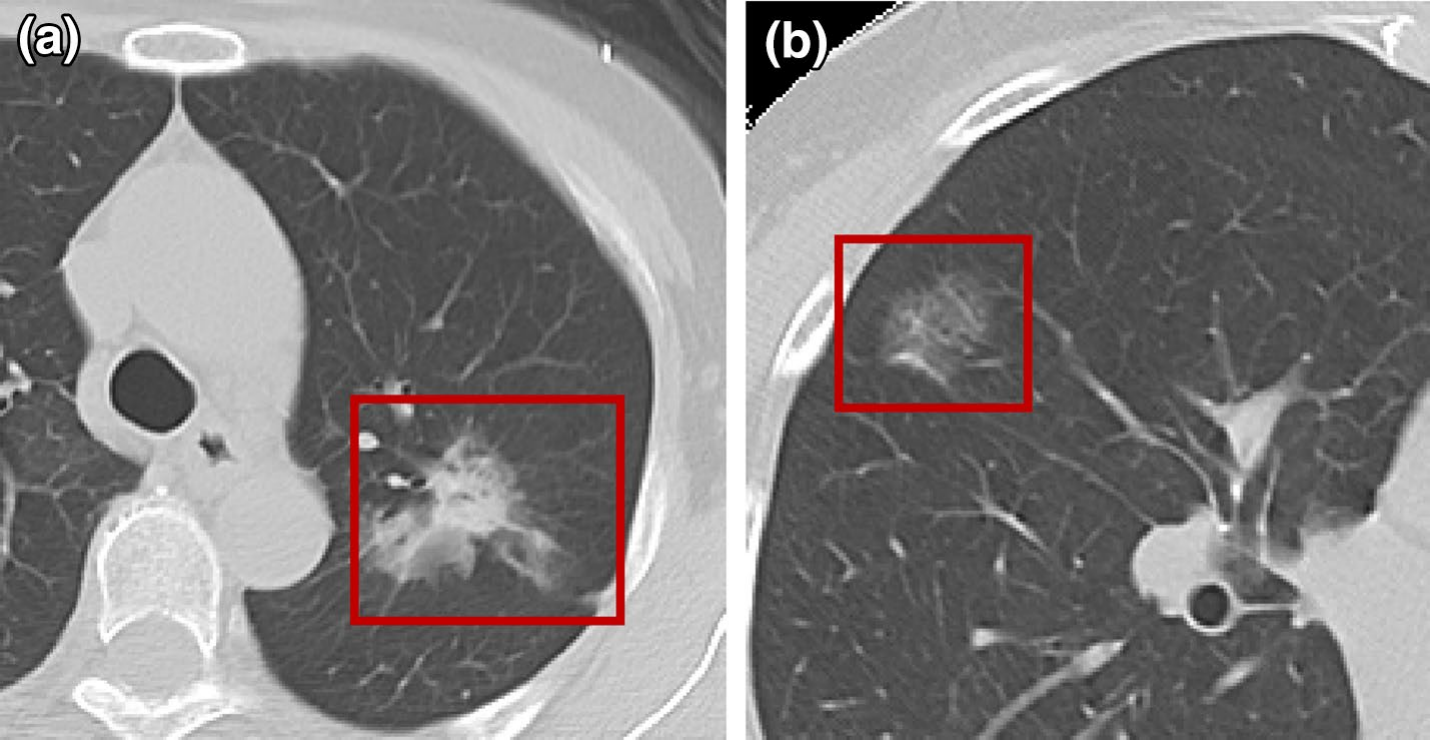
Pulmonary nodule on chest CT. a Thin-section CT with 3-mm collimation showing a 2.2-cm ground-glass dominant nodule at the upper left lobe. b Thin-section CT with 3-mm collimation showing a lobulated contour of the pure ground-glass nodule.
